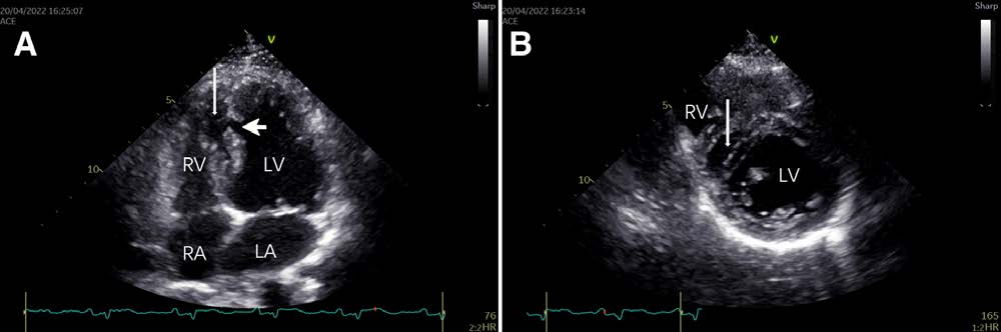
Two-dimensional (2D) echocardiography. (A) The apical 4-chamber view showed the bilayer structure of the ventricular septum (long arrow), and the continuity interruption in the left ventricular surface (short arrow). (B) The parasternal papillary muscle short-axis view clearly showed the bilayer structure of the ventricular septum (long arrow). LA = left atrium, LV = left ventricle, RA = right atrium, RV = right ventricle.
